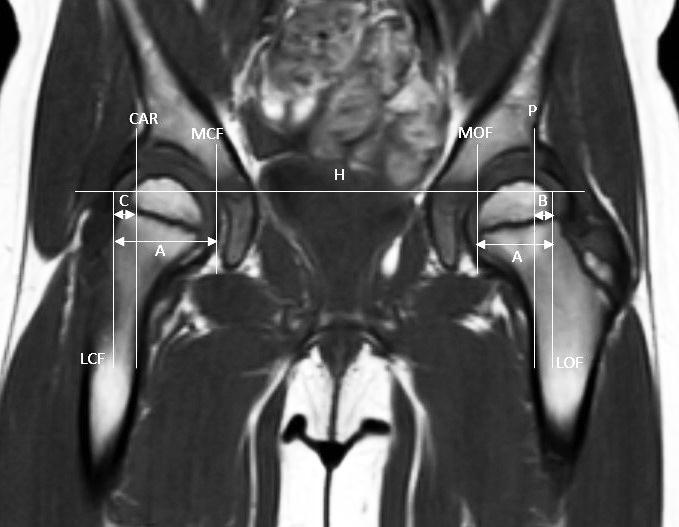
Coronal T1-weighted magnetic resonance imaging scan of pediatric hip included in this study. LOF: lateral edge of osseous femoral head, MOF: medial edge of osseous femoral head, LCF: lateral edge of cartilaginous femoral head, MCF: medial edge of cartilaginous femoral head, H: Hilgenreiner’s line, P: Perkin’s line, and CAR: vertical line through lateral edge of cartilaginous acetabular roof perpendicular to Hilgenreiner’s line. MP_MRI_ = B/AЧ100%, CMP = C/AЧ100%
